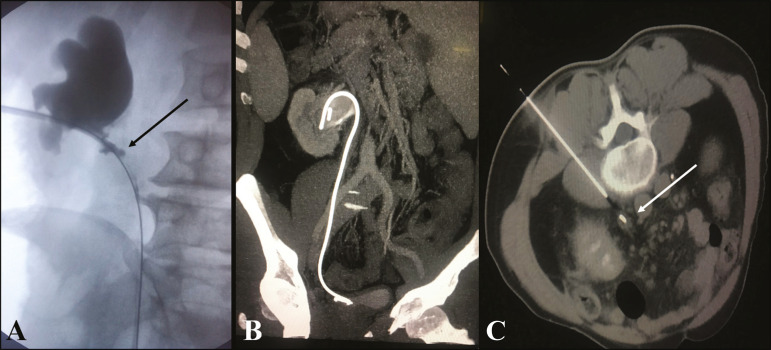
A: Pyelography performed before percutaneous insertion of the double J catheter showing stenosis of the proximal ureter with parietal irregularities (arrow). B: Post-contrast coronal abdominal CT scan, MIP reconstruction, showing the properly positioned double J catheter. C: CT-guided percutaneous biopsy with posterior access and coaxial technique showing ureter thickening with maintenance of the double J catheter for ureteral protection. Anatomical and pathological examination revealed an inflammatory pseudotumor of the ureter.
